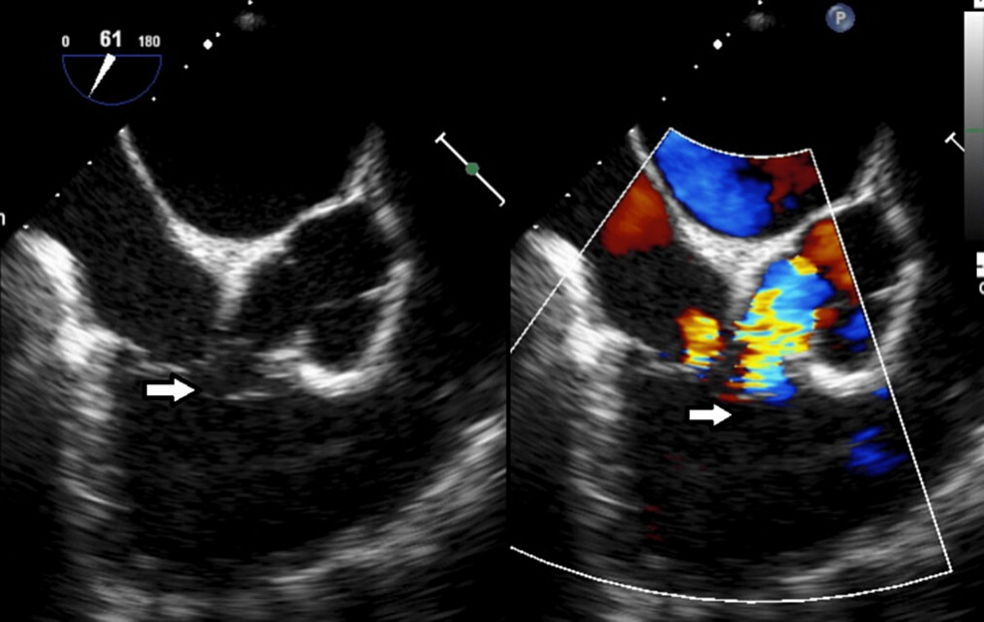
Transesophageal Echocardiogram (TEE). Mid-esophageal TEE color-compare still frame showing a short axis of the aortic valve and aortic root, with a ruptured non-coronary sinus of Valsalva aneurysm into the right ventricle with left-to-right shunting. The aorta-to-right ventricular jet enters the right ventricle just distal to the plane of the tricuspid valve. On the left (2D image without color), the arrow shows the SOVAR into the right ventricle. The right (color image) arrow shows the SOVAR shunting jet entering the right ventricle.
